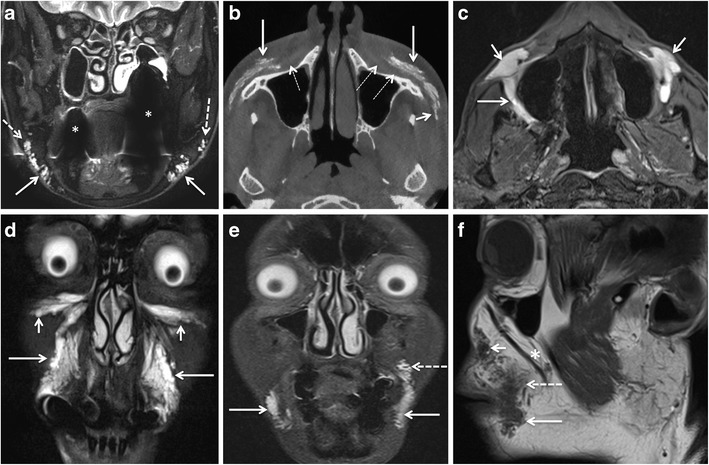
Commonly injected facial fat compartments and characteristic aspect of various fillers at MRI and CBCT. In all presented cases, the patients were symptom-free and were imaged for other reasons. a 55-year-old woman with collagen filler injections 5 months previously. On coronal STIR image, collagen filler injections in the superior (*dashed arrows*) and inferior (*arrows*) jowl compartments are seen as hyperintense, lobulated and reticulated areas. Asterisks indicate large artefacts due to dental implants. b 62-year-old woman with CHA injections 3 years previously. On axial CBCT with bone window settings, streaky calcific density of CHA filler is seen in the left and right medial and middle superficial cheek compartments (*arrows*) and extending towards the left lateral temporal cheek fat (*short arrow*). Thin dashed arrows point at the SMAS and muscles of facial expression (levator labii superioris, levator labii superioris aleque nasii, and zygomaticus minor muscles). c 64-year-old man with HIV-LA and HA injection 6 months earlier. Axial STIR image reveals HA injection performed in the deep medial compartment (*short arrows*) and buccal fat pad (*large arrow*). d 45-year-old woman with bilateral HA injections. On coronal STIR image, HA is detected in inferior orbital compartments (*short arrows*) and nasolabial fat compartments (*long arrows*). e and f 60-year-old woman with bilateral HA injections for cosmetic purposes. On coronal STIR image (e), HA injection of the superior (*dashed arrow*) and inferior jowl compartments (*arrow*). Sagittal T1 W image (f) shows hypointense HA in the nasolabial fat (*short arrow*), medial superficial cheek compartment (*dashed arrow*) and jowl compartment (*arrow*). Non-injected buccal fat compartment (*asterisk*)
